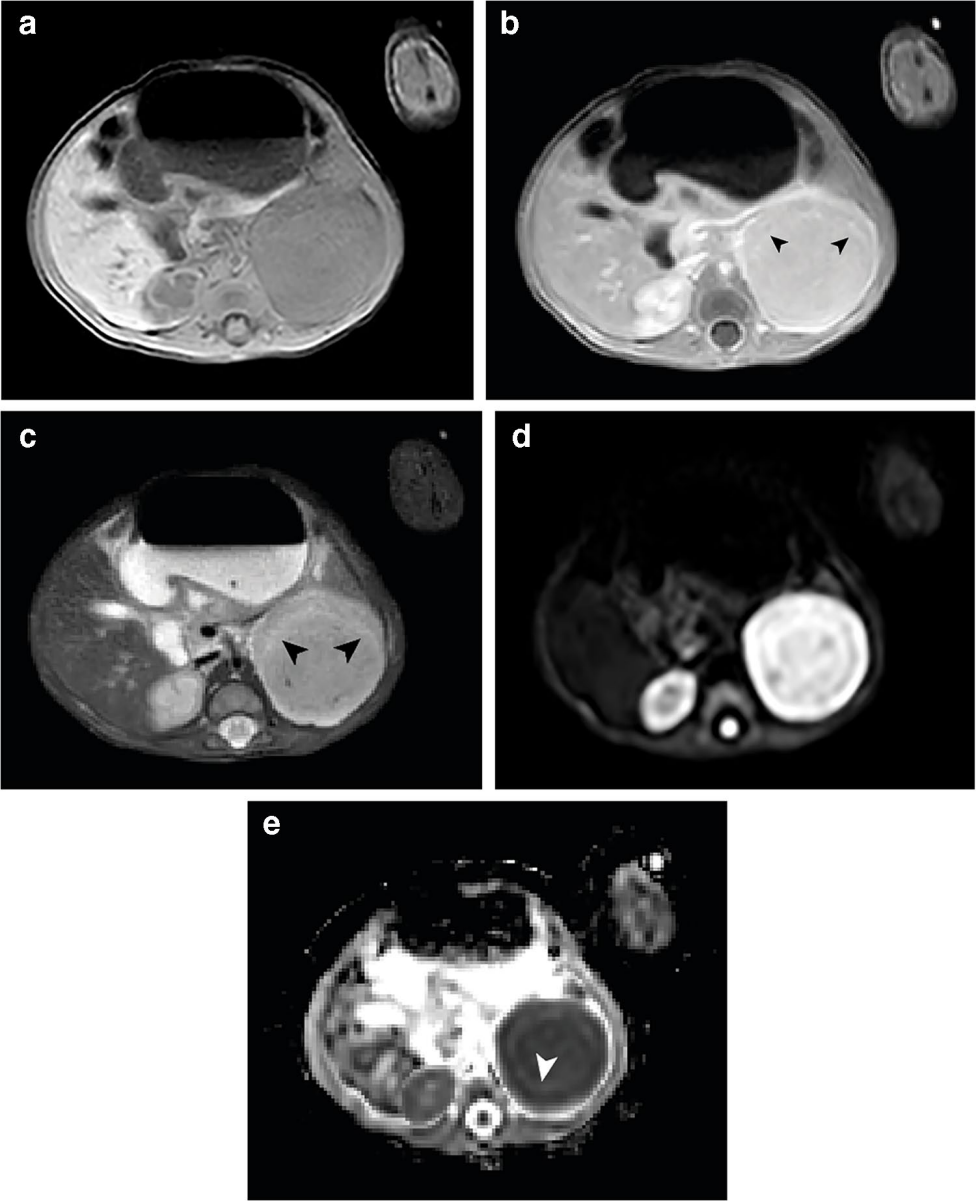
Magnetic resonance imaging of a 1-month-old boy with a left-sided classic type congenital mesoblastic nephroma with a volume of 44 cm^3^. a Axial T1-weighted image shows a homogeneous and isointense tumor. b Contrast-enhanced axial T1-weighted image shows homogeneous enhancement, with the concentric ring sign (*arrowheads*). c This homogeneity of the tumor as well as the hyperintense ring sign are also seen on the axial T2-weighted sequence, with an isointense appearance (*arrowheads*). d On the axial diffusion-weighted imaging b1000 sequence the tumor shows a homogeneous high intensity. e On the calculated axial apparent diffusion coefficient map, the tumor shows no evident increased diffusion restriction compared to the healthy contralateral renal tissue, with a median apparent diffusion coefficient value of solid parts of the tumor of 1.100×10^−3^ mm^2^/s. A hyperintense concentric ring is observed (*arrowhead*)
